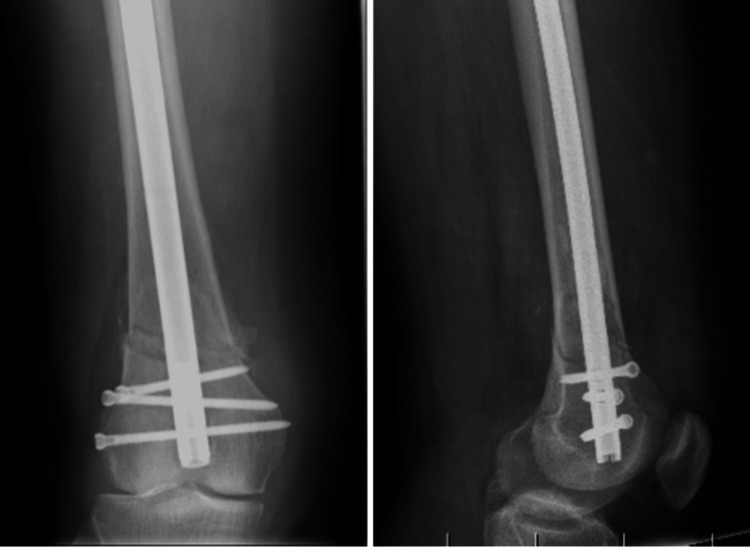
Case 5 follow-up X-ray at six months
